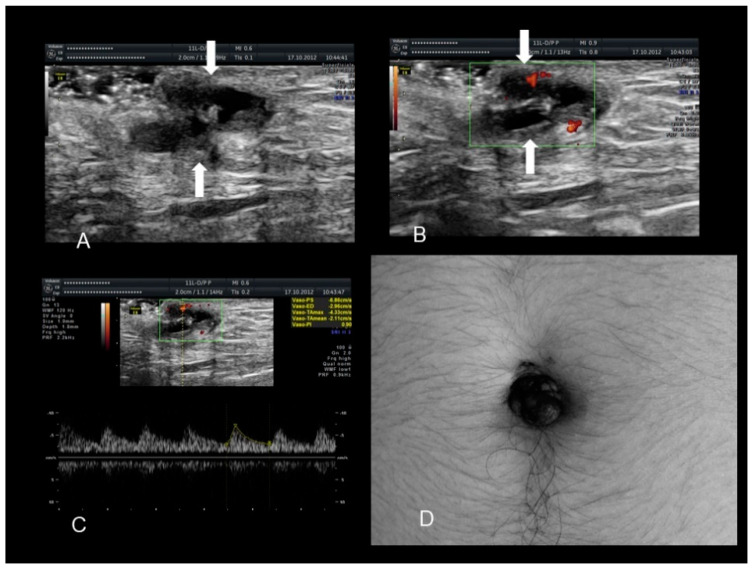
The ultrasonographic appearance of a Villar’s nodule (see white arrows) using B-mode (A) and color Doppler (B,C) with some color spots due to peripheral vascularization in a woman without previous abdominal surgery. In this case the ultrasonographic appearance was more cystic than solid (A–C). The nodule at visual evaluation (D).
